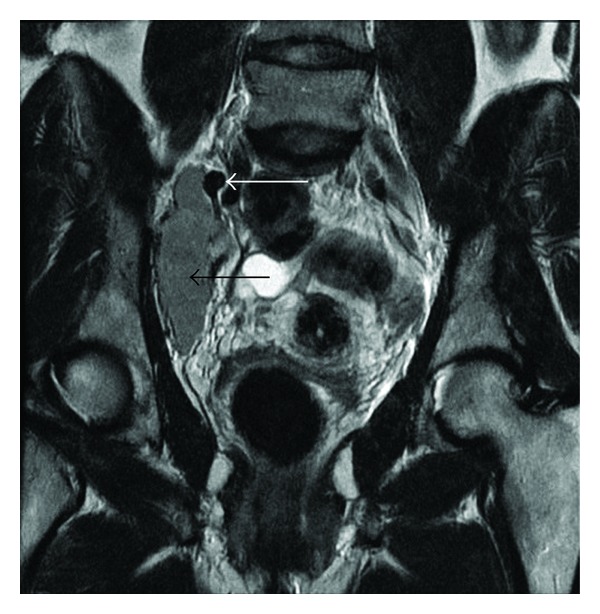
Pelvic magnetic resonance imaging: coronal T2-weighted imaging shows a well-capsulated, hyperintense, solid mass (black arrow), next to the iliac vessels (white arrow).
